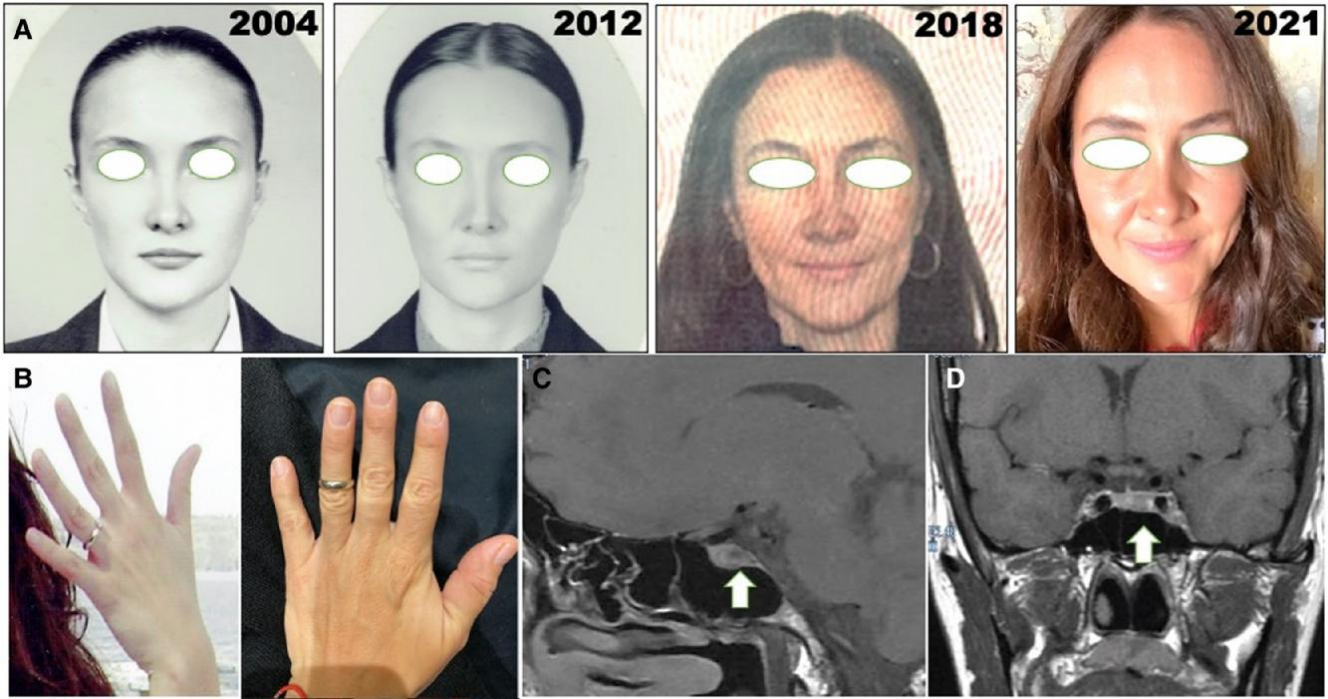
A. Mild clinical changes in the patient over time with prognathism, frontal bone bossing, a wider nose, and increased face skin folds apparent in photographs. B. Failure to fit the wedding ring at diagnosis in comparison to past. C. Microadenoma identified with T1 gadolinium-enhanced MRI sequence in the middle/center of the pituitary (white arrow). D. Coronal T1 gadolinium-enhanced MRI sequence showing the microadenoma (white arrow).
